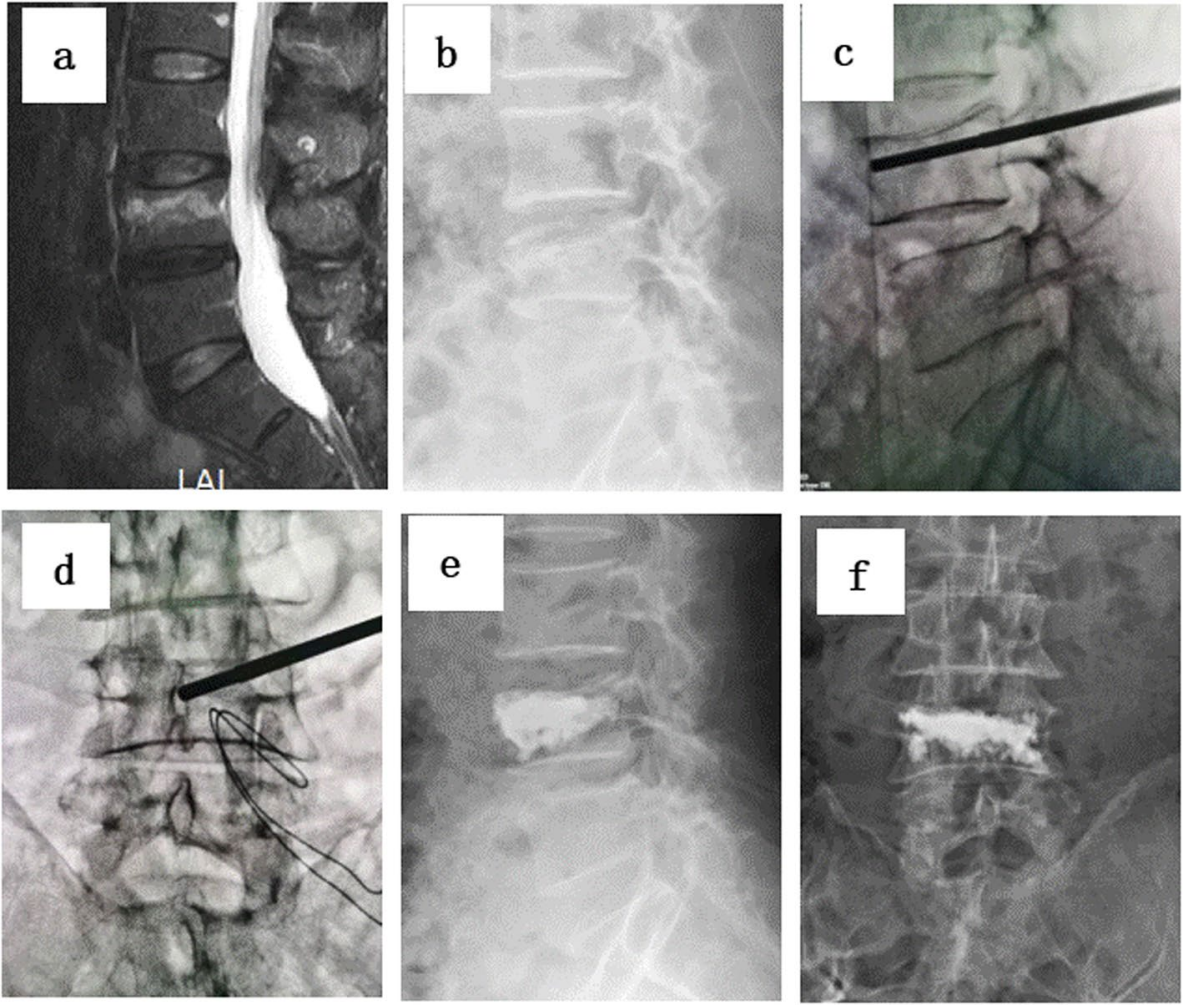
a-f Percutaneous kyphoplasty for the treatment of a L4 vertebral compression fracture in unipedicular approach. a-b Preoperative lateral radiograph and MRI showing a L4 vertebral compression fracture. c-d intraoperative view (e-f) Postoperative lateral radiograph showing cement distribution after undergoing PKP surgery of L4 vertebral compression fracture
